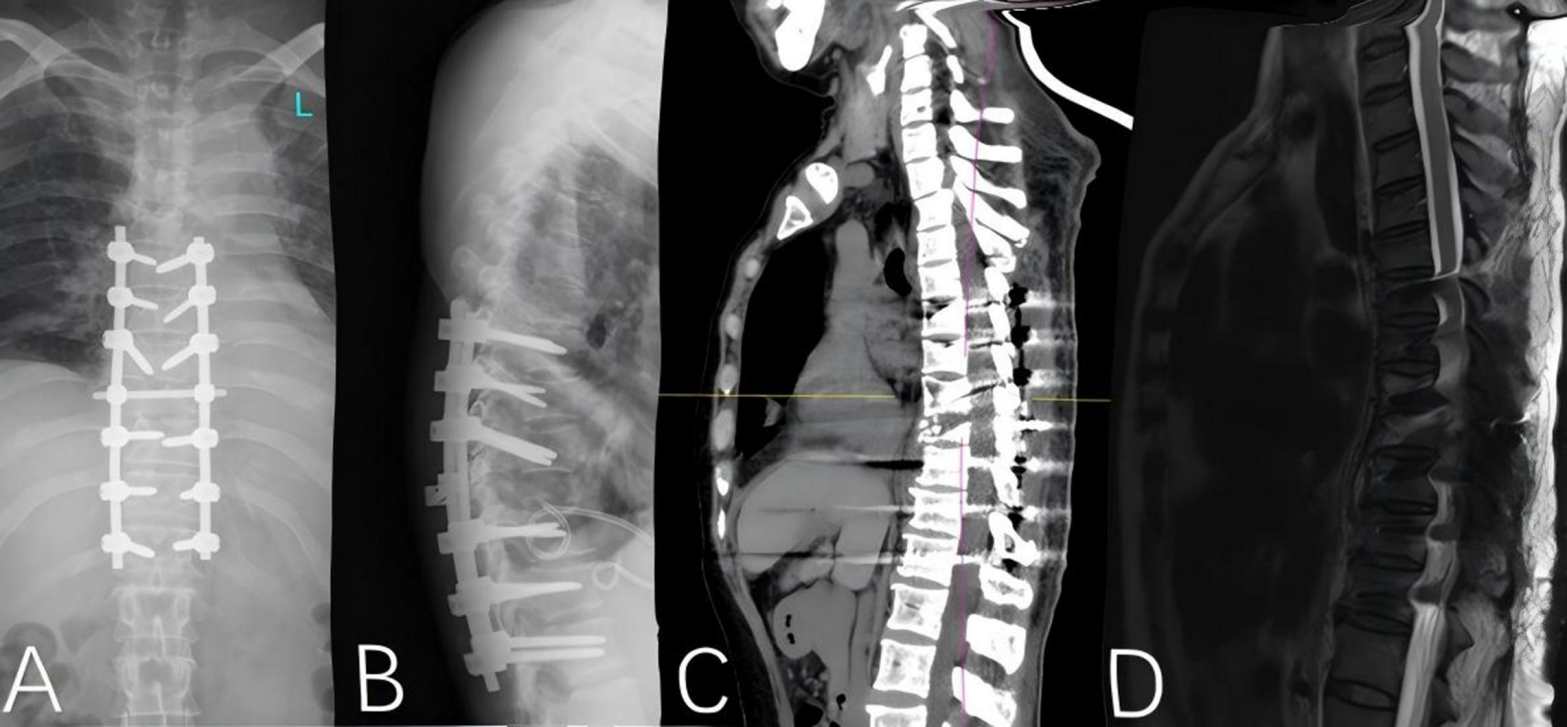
Postoperative digital radiographs of the spine, including (A) anteroposterior and (B) lateral views, demonstrate successful correction of the thoracolumbar deformity. The internal fixation hardware is well-positioned, and the physiological curvature of the spine is largely restored. Computed tomography (CT) and magnetic resonance imaging (MRI) scans (C,D) confirm satisfactory reduction of the fractured vertebral body.
